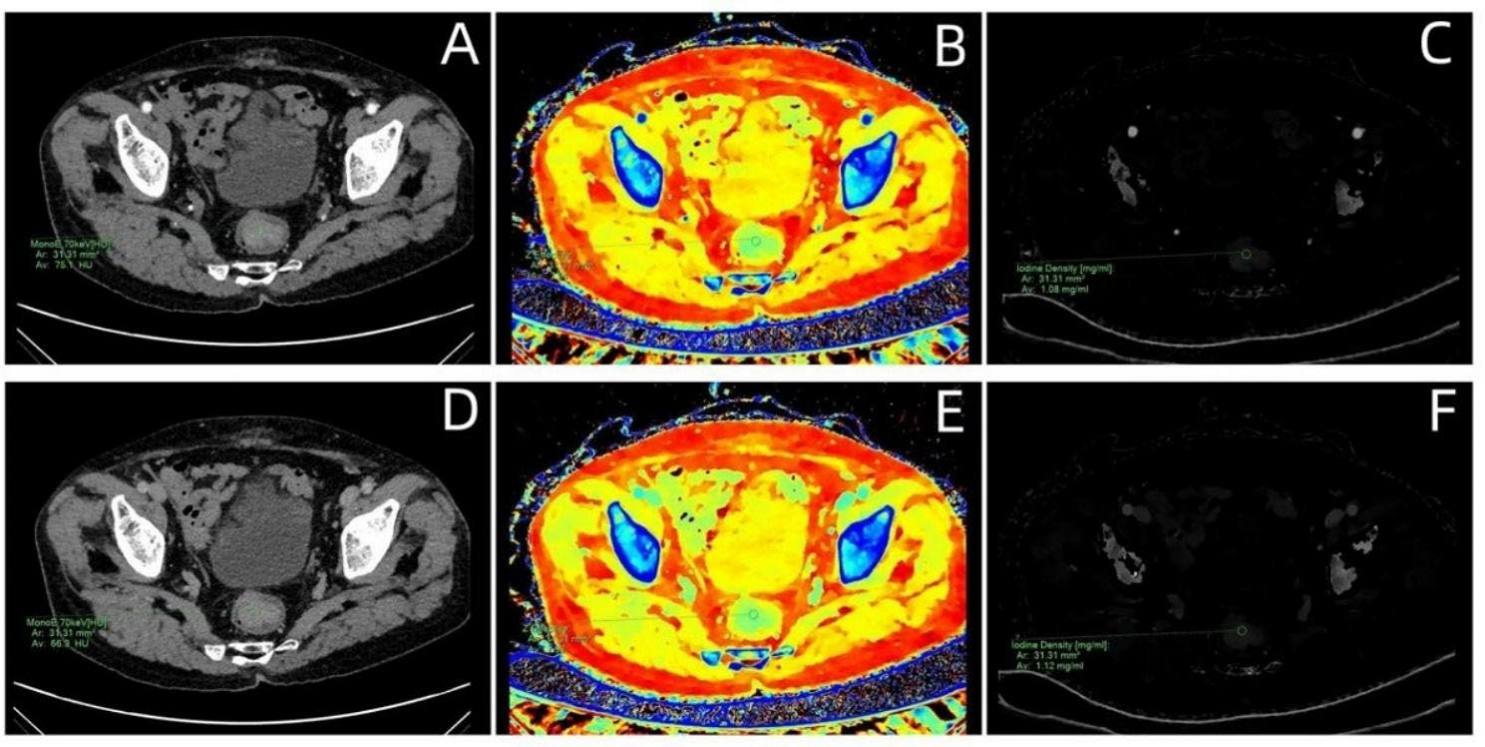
A man with colon cancer.DESCT parameters including monochromatic 70 CT value,iodine concentration (IC), and effective atomic number were measured with the same region of interest at the same location.The figure [A-C] shows the arterial phase,and the figure [D-F] shows the venous phase
